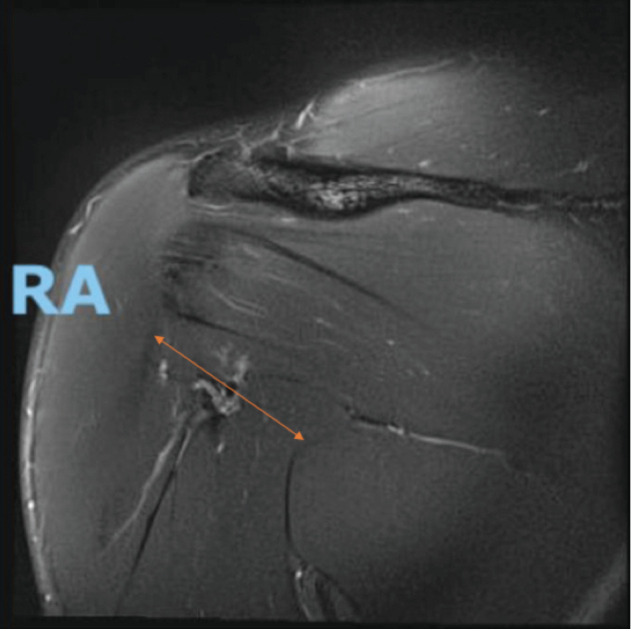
MRI coronal view of right chest showed complete PMT rupture and medialisation of muscle belly. Lower PM is retracted and leaving a 5cm gap.
